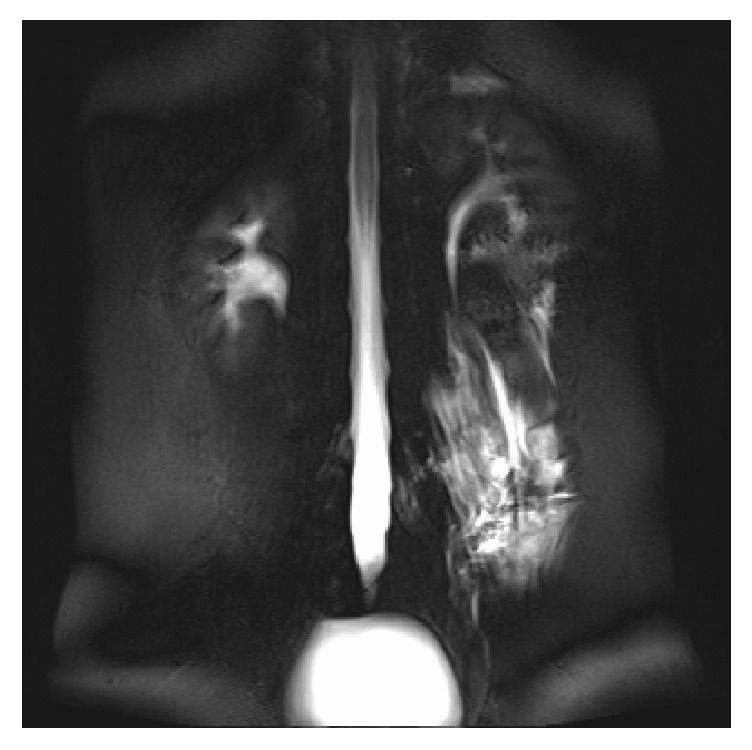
The MRI myelogram shows an abnormal fluid signal intensity in the left lumbar area, along the left paraspinal muscle and soft tissues at the L3–4–5 level, probably due to CSF leakage.
